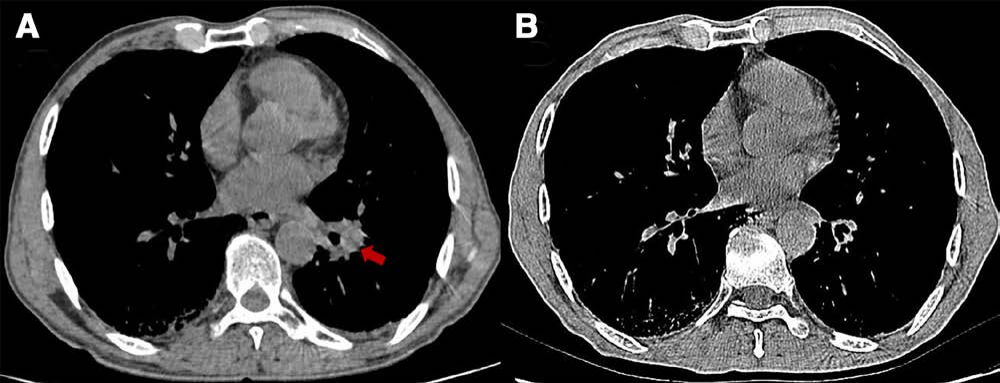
(A) CT scan on June 9, 2023, showing multiple nodules in the lower lobe of the left lung, the largest of which was approximately 3 cm in diameter, with spiculated edges (indicated by the red arrow). (B) CT scan on November 13, 2024, showing significant shrinkage of the nodules in the lower lobe of the left lung after treatment, with marked improvement in the patient’s cough and dyspnea. CT = computed tomography.
